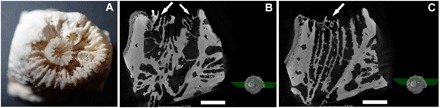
3D computed tomography sections of a *C. caespitosa* calix showing three new calices. (A) Photograph of the scanned corallite. (B and C) Arrows point to the new calices, which show connections to the skeletal structures of the original polyp. Scale bars: 0.15 cm (photo credit: D. K. Kersting, Freie Universität Berlin).
